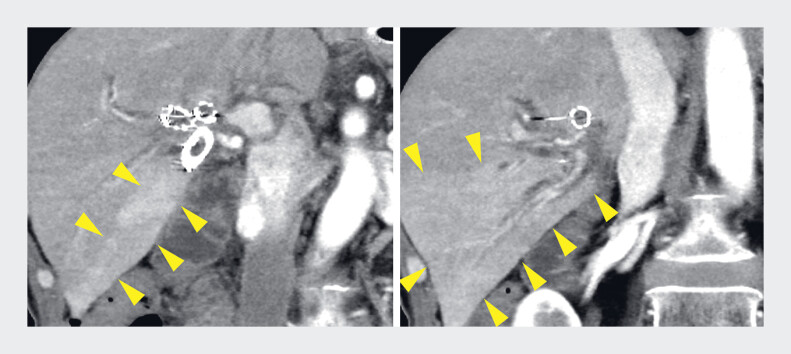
Contrast-enhanced computed tomography (CT) shows dilation of the B6 bile duct and increased enhancement of the posterior segment (arrowheads).
